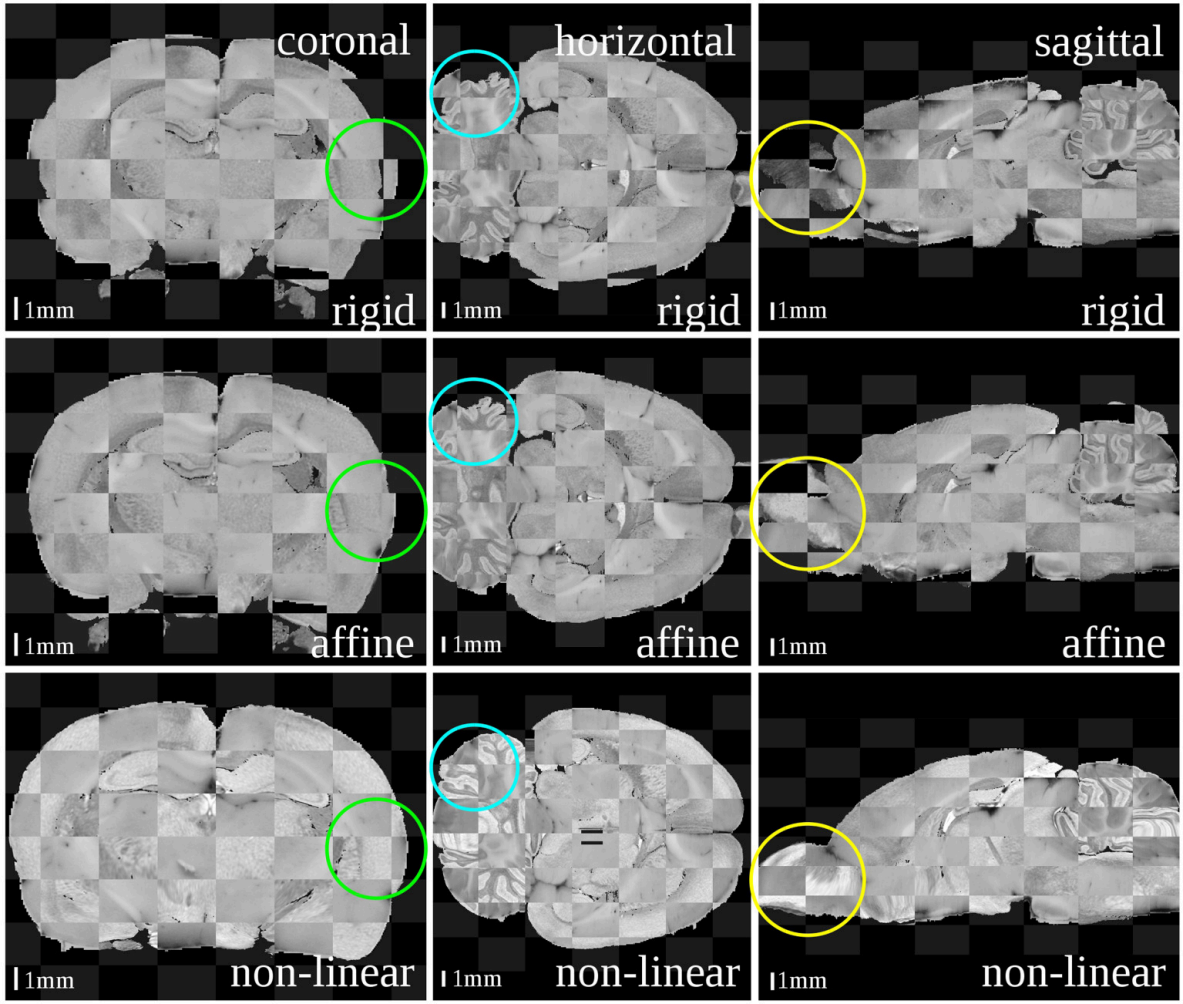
Checkerboard images of the blockface volume of the receptor brain and the T2^*^-weighted atlas MRI template after each registration step: rigid (first row), affine (second row) and non-linear (third row). Considering size and shape of the brain and especially its outer surface (green circles), cerebella (blue circles) and olfactory bulbs (yellow circles) the matching quality increased with increasing degree of freedoms of the transformations.
